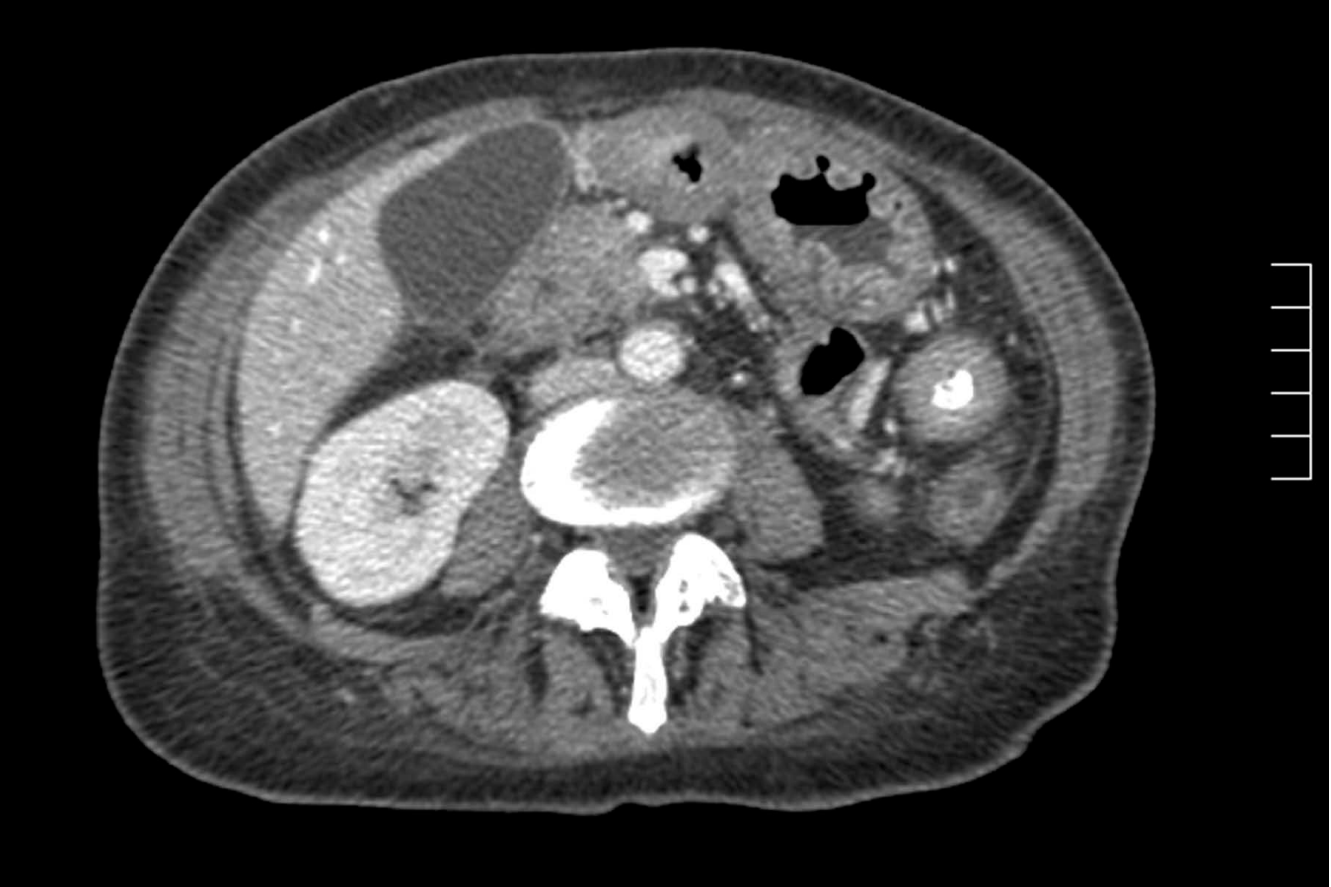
CT scan demonstrating thickening of colonic wall with narrowing of lumen.
